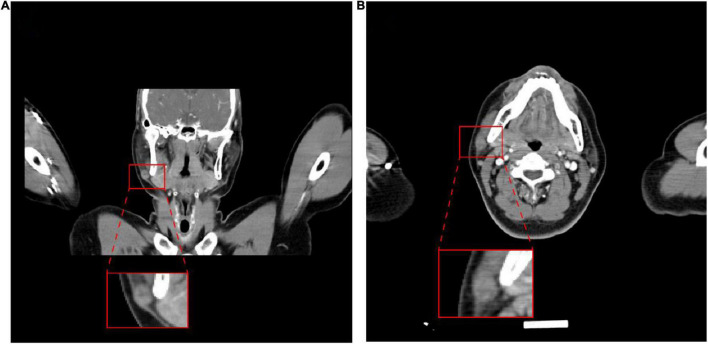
Images of the tumor. Computed tomography showed a nodule on the lower right side of the parotid gland. (A) Coronal plane; (B) transverse plane.
